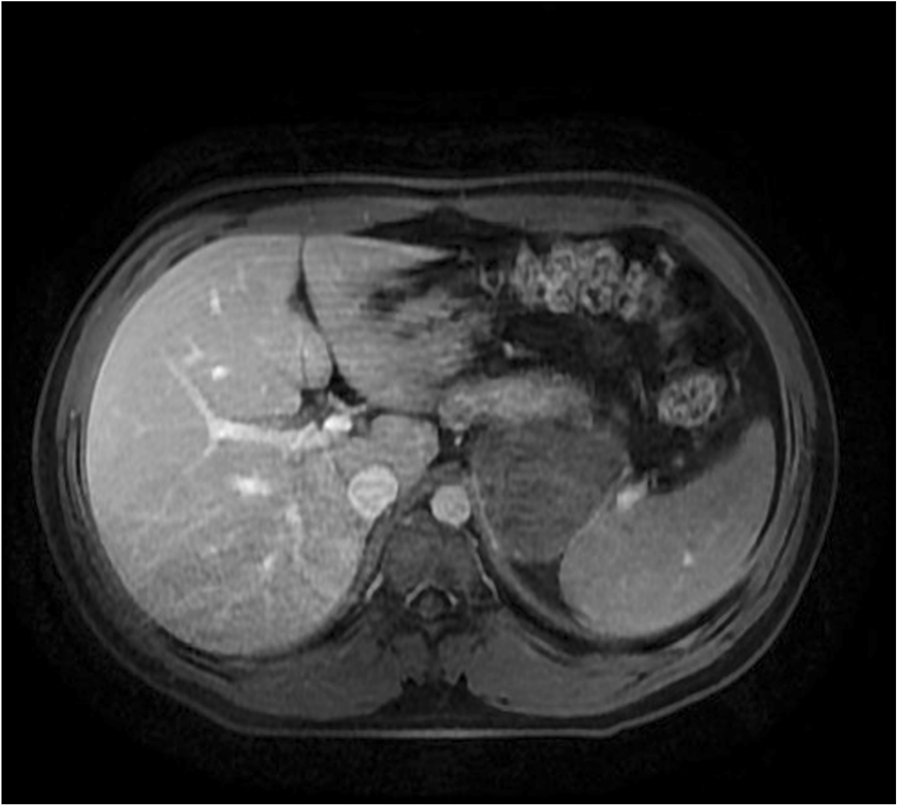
Axial T1-weighted gadolinium contrast-enhanced magnetic resonance image shows the larger cystic mass with peripheral enhancement
